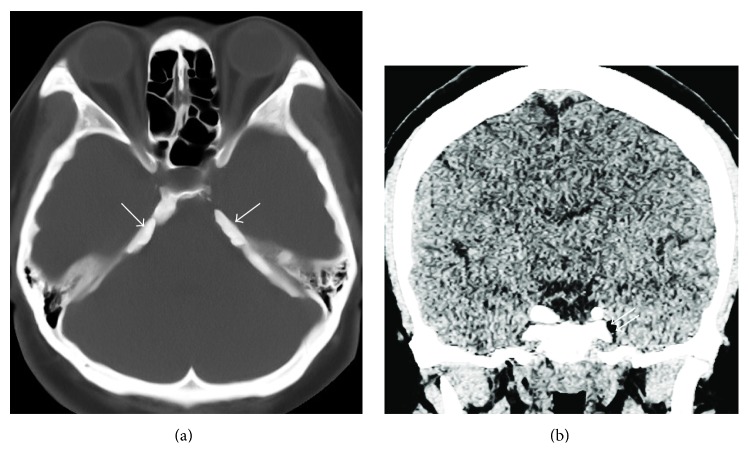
Brain CT image shows plaque-like tentorial ossification (arrow) on bone window (a) and an air bubble (double arrows) adjacent to left Meckel's cave on brain window (b).
